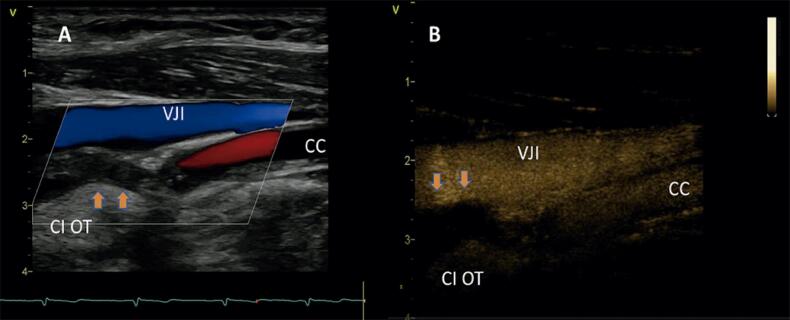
Oclusão da carótida interna. A) Ausência de fluxo na artéria carótida interna ao Doppler colorido; B) Contraste ultrassonográfico não visualizado na luz da carótida interna ocluída.
